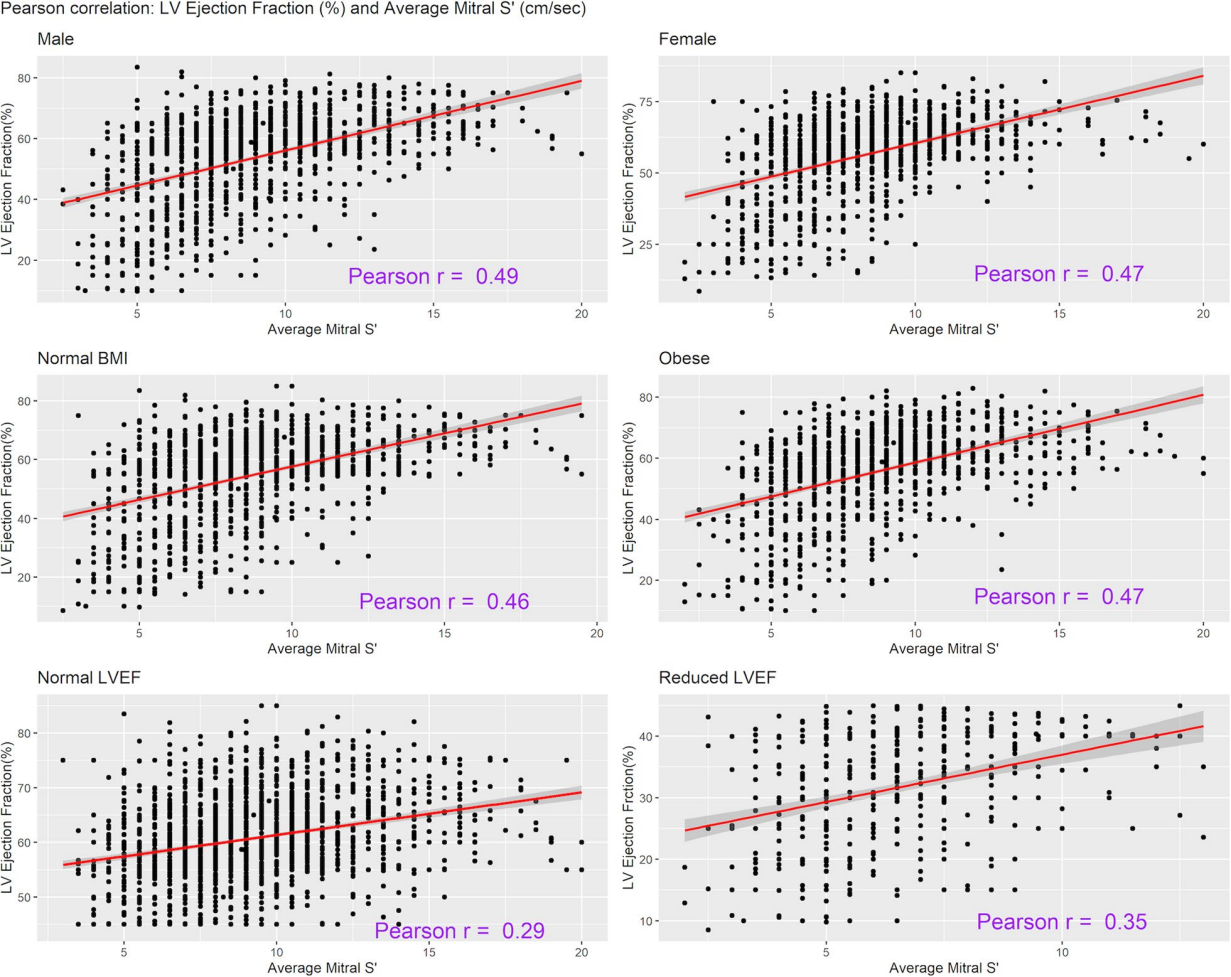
Pearson correlation: left ventricle ejection fraction (LVEF %) and average Tissue Doppler-Derived Left Ventricular Systolic Velocity (mitral S’) (cm/s) in with sub-group analysis based on gender, BMI (BMI < 30 kg/m^2^ or ≥ 30 kg/m^2^), and normal or reduced LVEF (LVEF < 45% or LVEF ≥ 45%)
